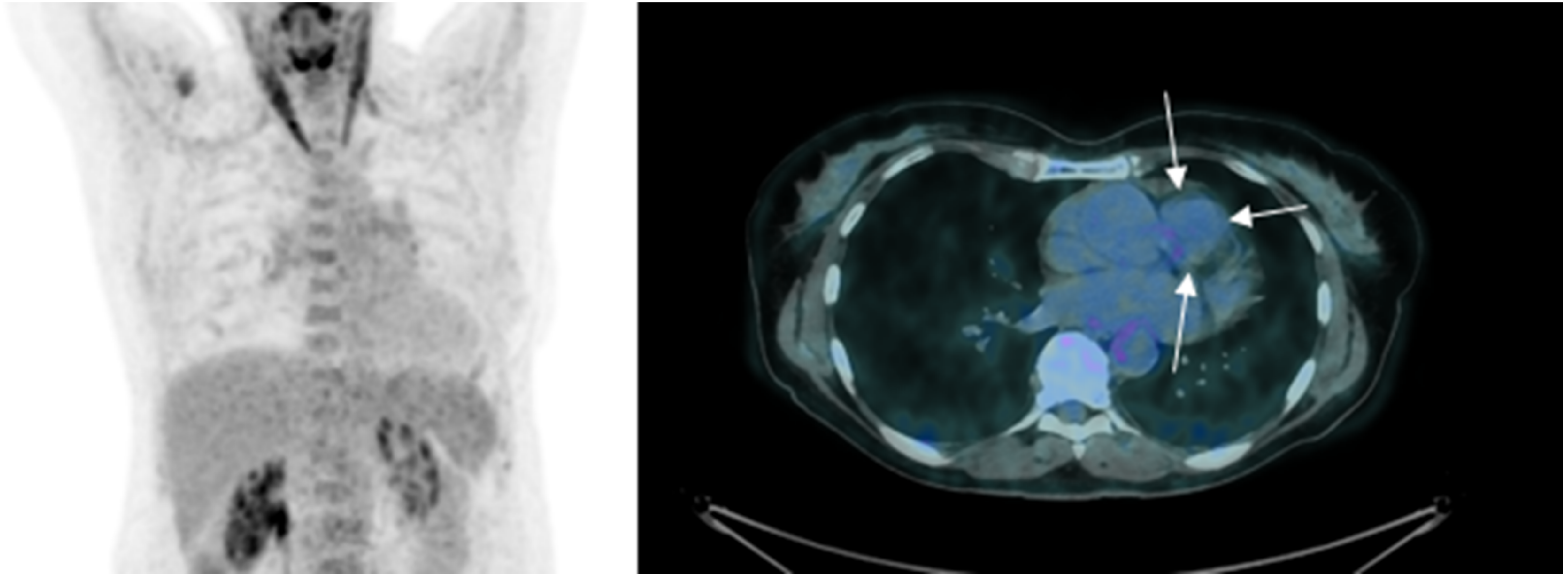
18-FDG PET/CT 2014—initial PET imaging reveals a low metabolic activity of the tumor (indicated by white arrows), comparable to that of the mediastinal blood pool [with a maximum standardized uptake value (SUVmax) of 2.6 for the tumor vs. 2.4 for the blood pool]. Please note that myocardial glucose metabolism is suppressed due to a diet low in carbohydrates and high in fats in the days leading up to the examination.
